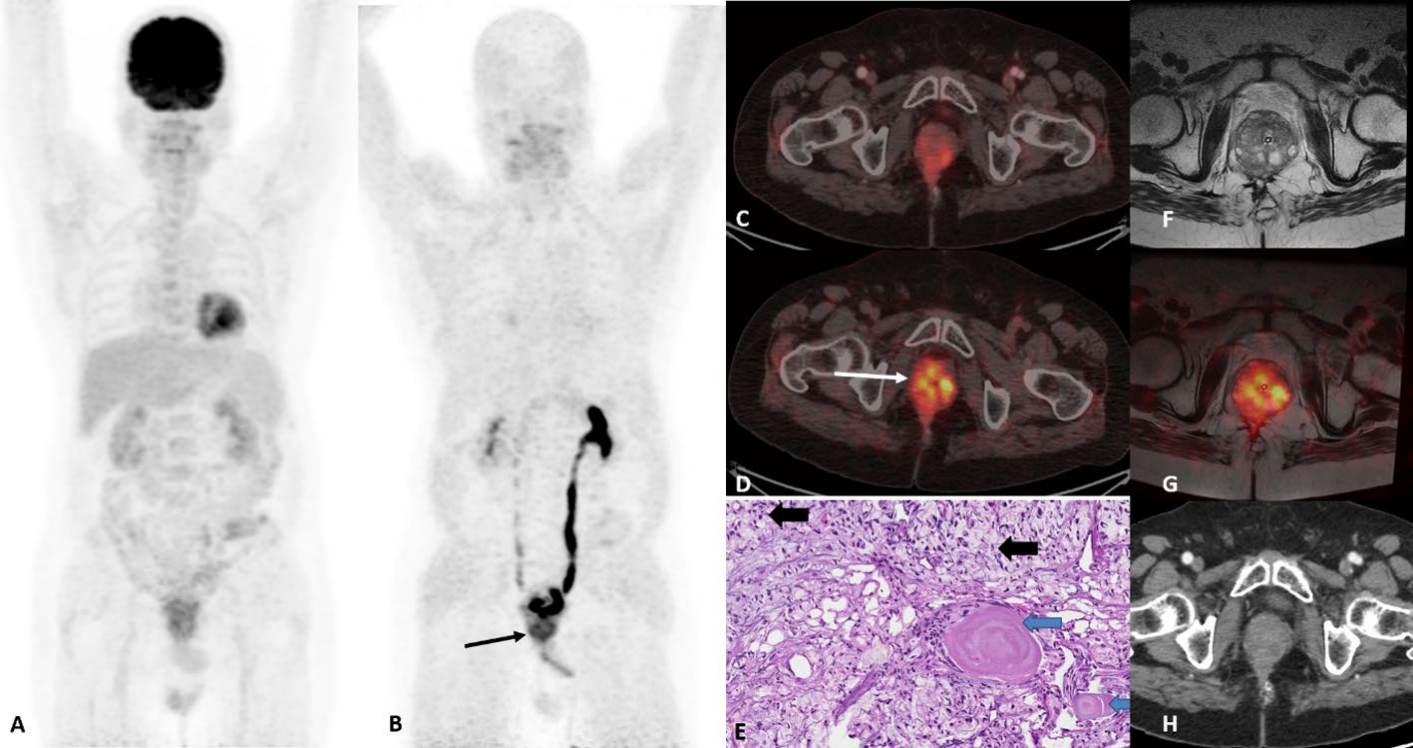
A FDG MIP, B MIP image of FAPI shows focus of uptake below the bladder (slender black arrow). C and D are axial fused images of FDG and FAPI PET-CT, respectively. The fused axial images of FAPI clearly show increased uptake (SUVmax-6.8) in prostate compared to FDG which shows only mild uptake. E H & E at 200× magnification image revealed normal prostate gland with corpora amylacea highlighted by blue arrows and tumour cells with signet ring morphology highlighted by bold black arrows, thus confirming the prostatic involvement by signet cell adenocarcinoma. F axial MRI and G FAPI MRI fusion images which show involvement of prostate. Seminal vesicles and base of bladder were also involved; (not shown H) contrast-enhanced diagnostic CT image which shows no significant abnormality
